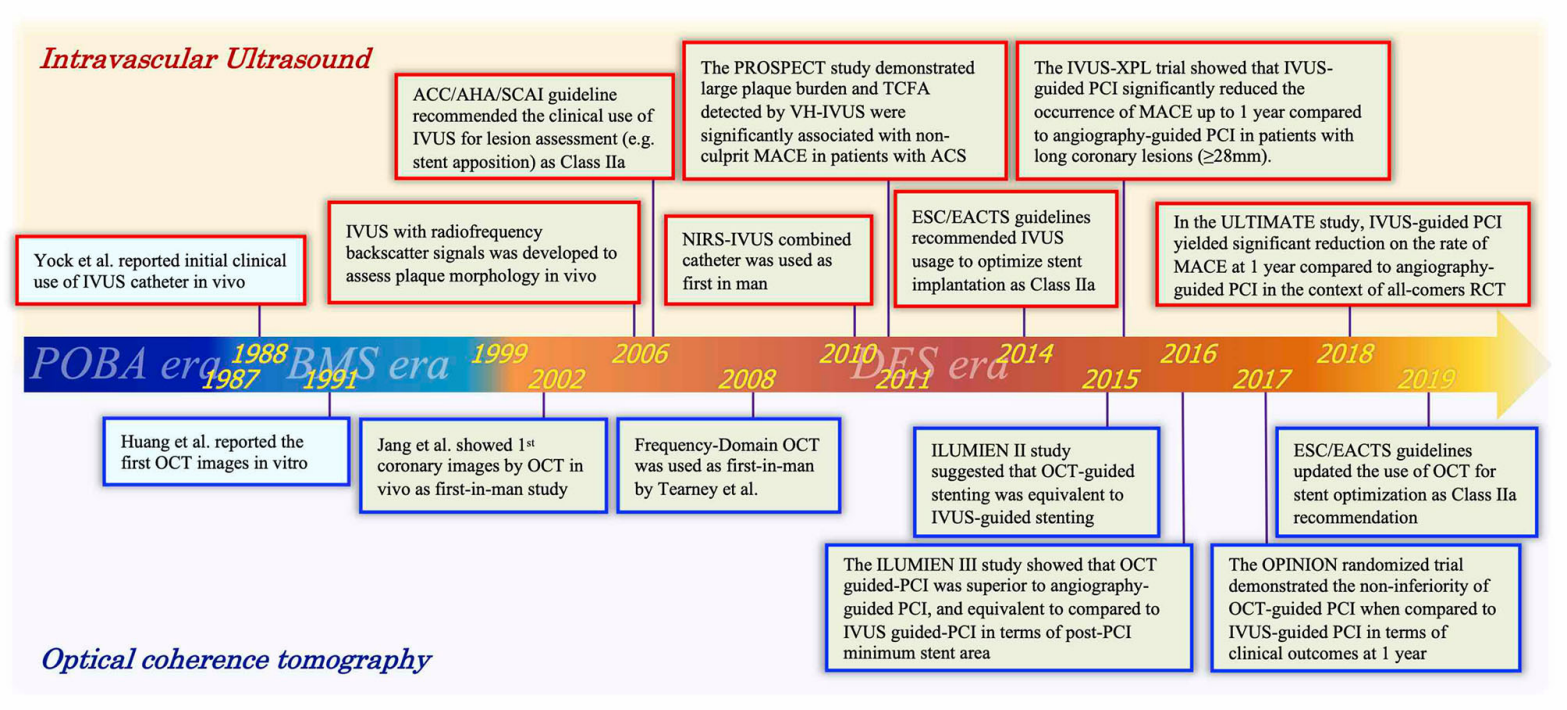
Timeline of intravascular ultrasound and optical coherence tomography with advances of PCI era. A history focused on intracoronary imaging devices (IVUS and OCT). Red and blue frames indicate representative events associated with IVUS and OCT, respectively. IVUS, intravascular ultrasound; OCT, optical coherence tomography; POBA, plain old balloon angioplasty; BMS, bare-metal stent; DES, drug-eluting stent; PCI, percutaneous coronary intervention; ACC, American College of Cardiology; AHA, American Heart Association; SCAI, Society for Cardiovascular Angiography; ESC, European Society of Cardiology; EACTS, European Association for Cardio-Thoracic Surgery; MACE, major adverse cardiac events.
